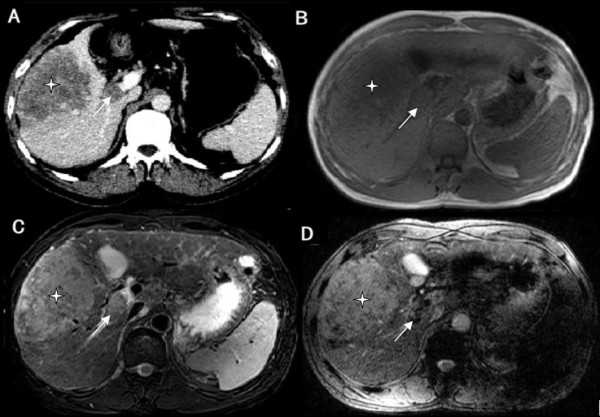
Bland thrombosis of the portal vein in a patient with hepatocellular carcinoma (HCC). A: Contrast-enhanced multidetector computer tomography (CE-CT); B: T1-weighted imaging (T1WI); C: T2-weighted imaging (T2WI); and D: Susceptibility-weighted imaging (SWI). HCC (*) is seen occupying the right lobe of the liver. A filling defect is noted in the right portal vein (white arrow), which exhibits lower signal intensity (SI) than the tumor by SWI.
